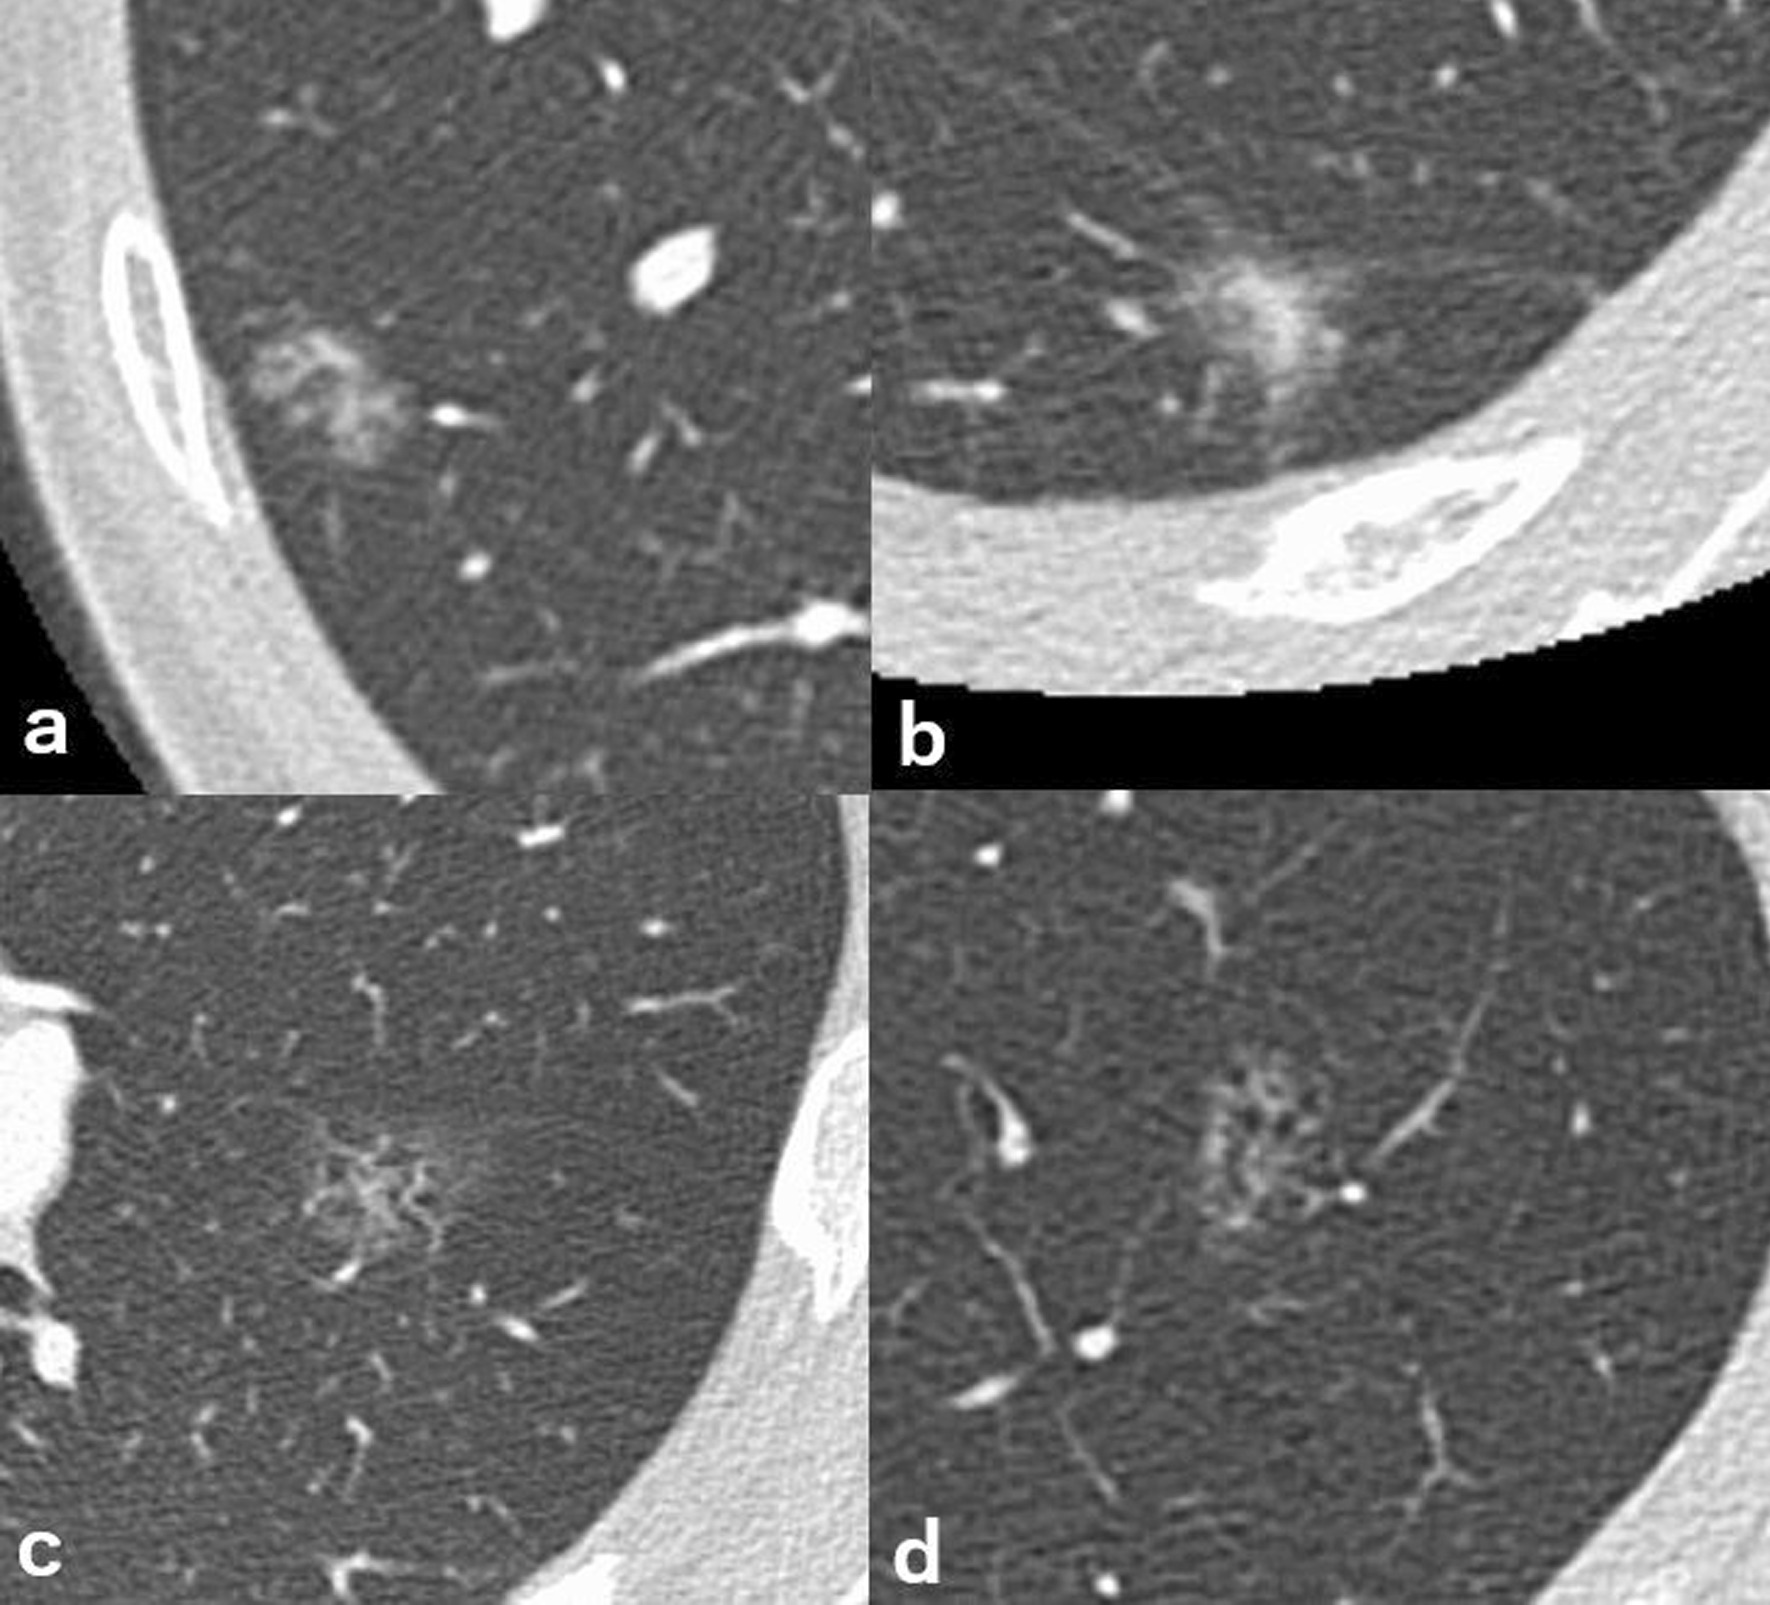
a A non-smoker’s MIA with 3 mm invasiveness. b A non-smoker’s MIA with 5 mm invasiveness. c A smoker’s MIA with 2 mm invasiveness. d A smoker’s MIA with 5 mm invasiveness. CT values tended to be lower in lesions arising from smoker’s lungs
